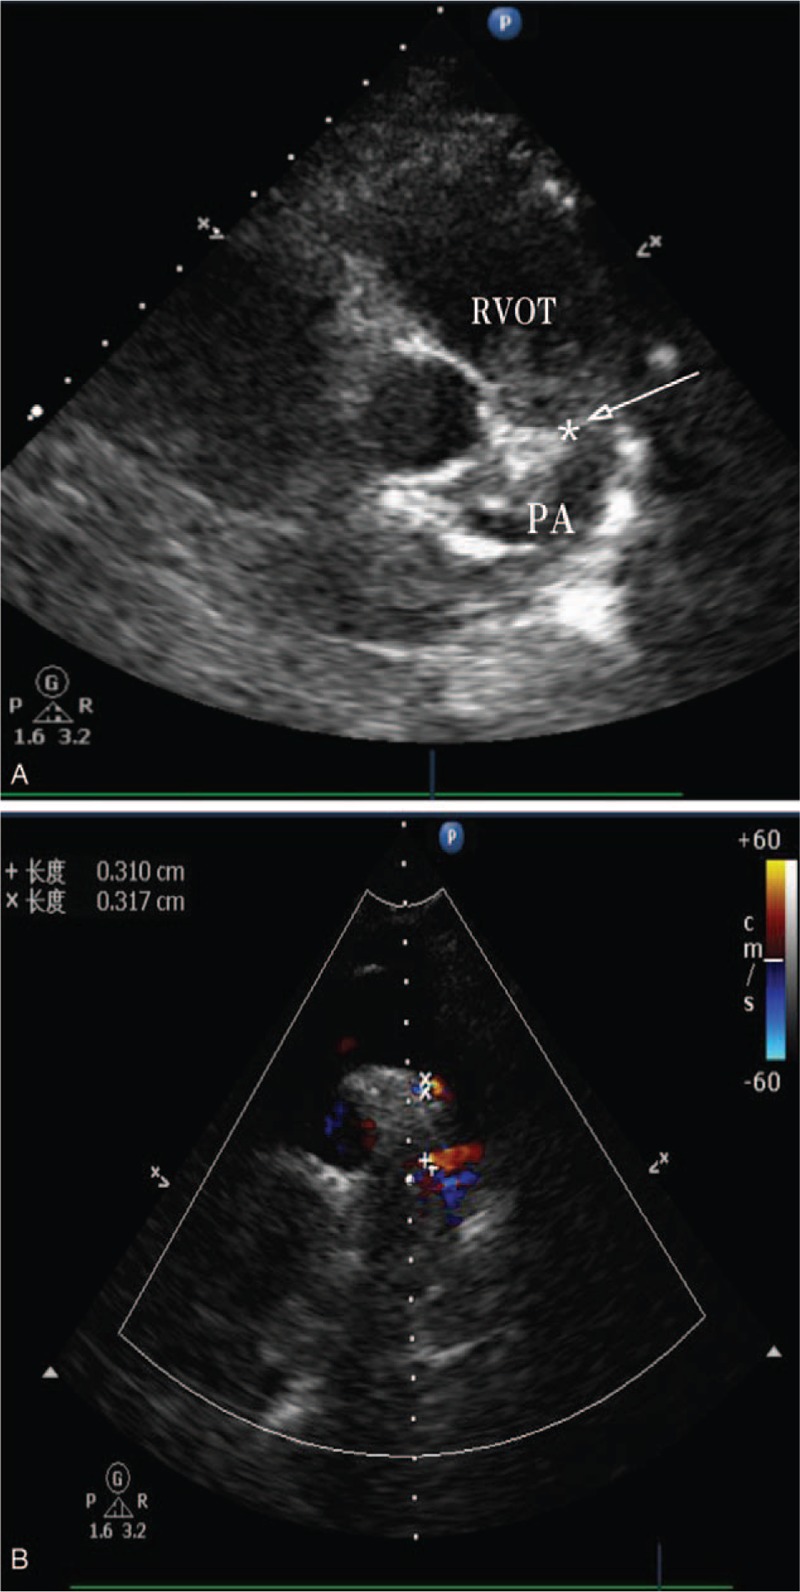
A: Transthoracic echocardiogram (parasternal short-axis view)shows the hypoechoic mass (asterisks)in the main pulmonary artery, extending down to the right ventricular outflow tract. RVOT=right ventricular outflow tract. B: The Color Doppler imaging demonstrates the presence of blood flow signals (asterisks) between the aorta and the pulmonary artery.
